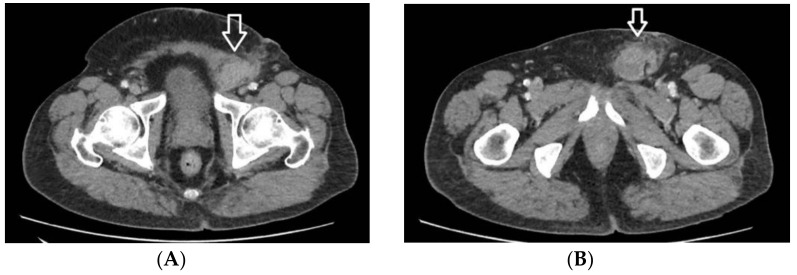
Abdominal CT image of the tumor (marked with white arrow) at different levels (A,B).
